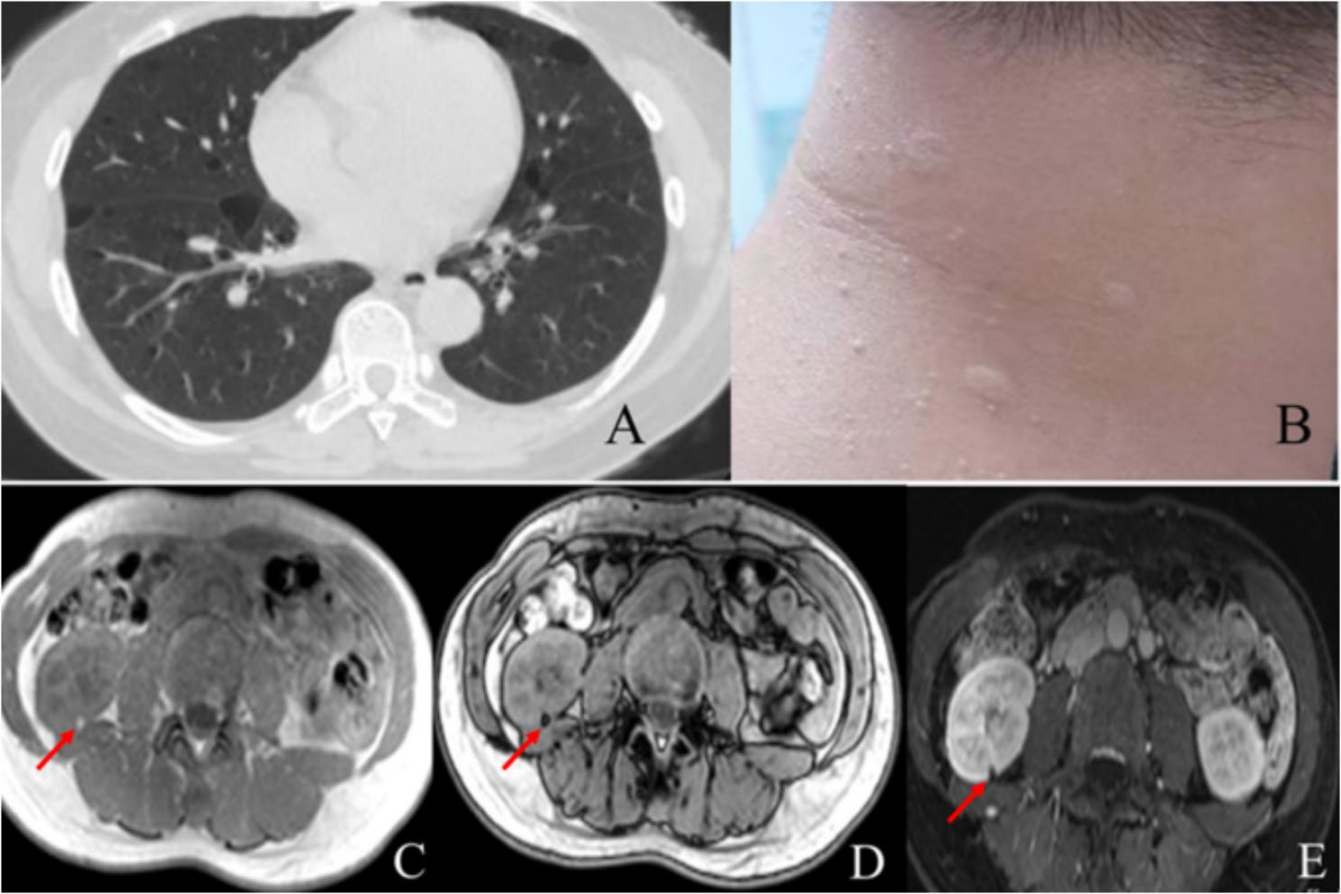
A:CT of patient 1 (44-year-old female) of family 8 shows multiple cysts distributed in the lower lung. B: Patient 1 (35-year-old male) of family 52 had fibrofolliculomas on the face and neck. C, D, E: Magnetic resonance imaging of the kidneys showing angiomyolipoma (patient 1 of family 12):C, focal abnormally high signal in the right kidney. D, decreased signal in the out of phase lesion suggesting adipose tissue. E, no enhancement on enhanced scan also suggests adipose tissue
